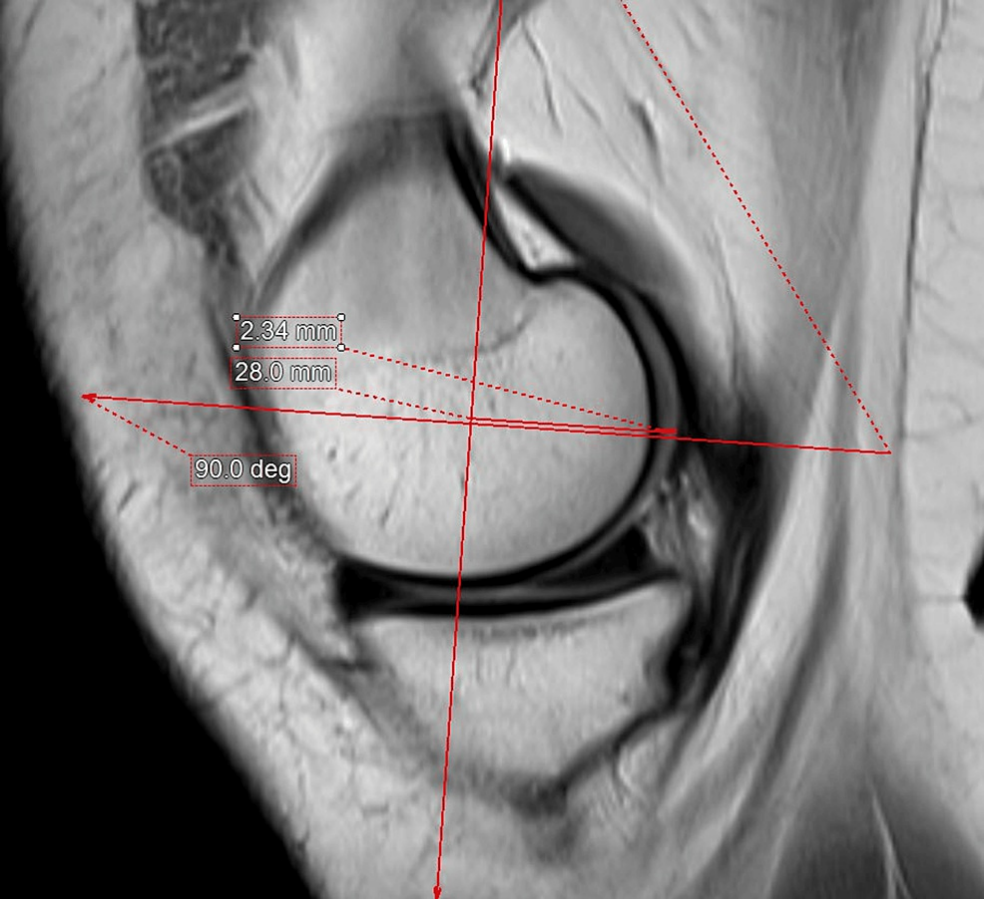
Sagittal proton-density MRI of the knee at the deepest part of the medial femoral condyle. The midsagittal axis is translated medially to measure the posterior condylar offset and posterior condylar cartilage thickness at the deepest part of the posterior condyle perpendicular to the drawn femoral posterior cortical axis.
